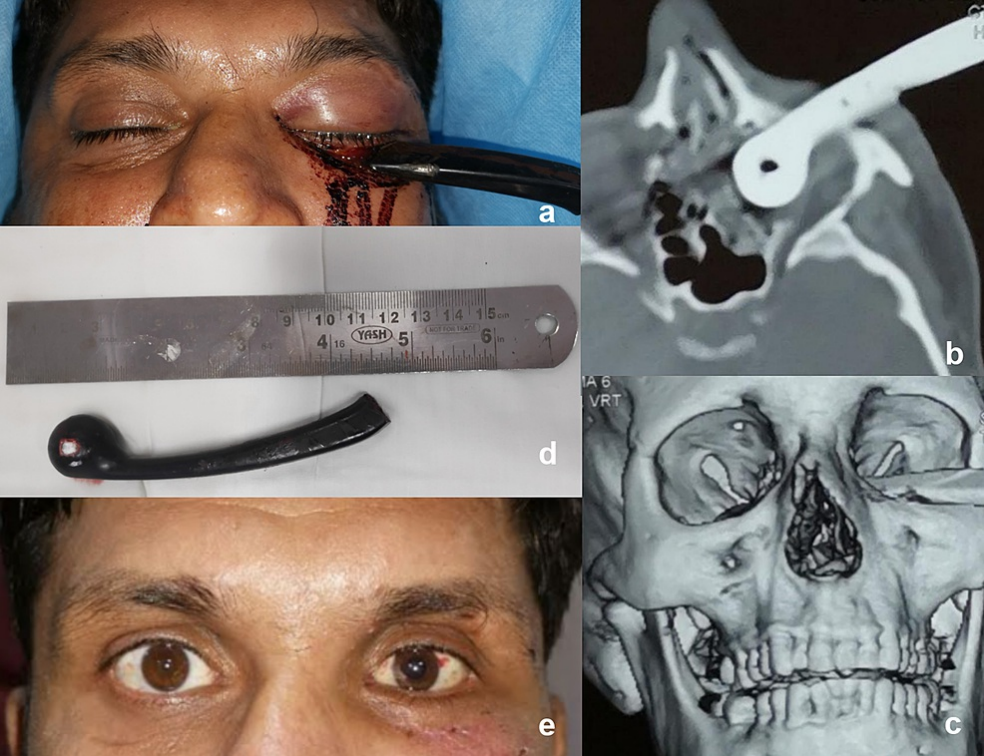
(a) Clinical photograph of the patient at presentation. (b) CT scan showing a well-defined hyperdense foreign body in the left orbit. (c) 3D reconstructed CT scan image showing a large foreign body in the left orbit. (d) Picture of the foreign body measuring 10.3cm x 2cm. (e) Clinical photograph of the patient after three weeks.
